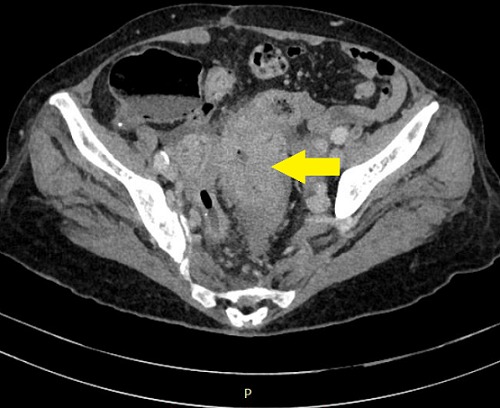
Abdominal computed tomography scan showing thickening of the sigmoid colon (indicated by arrow)
